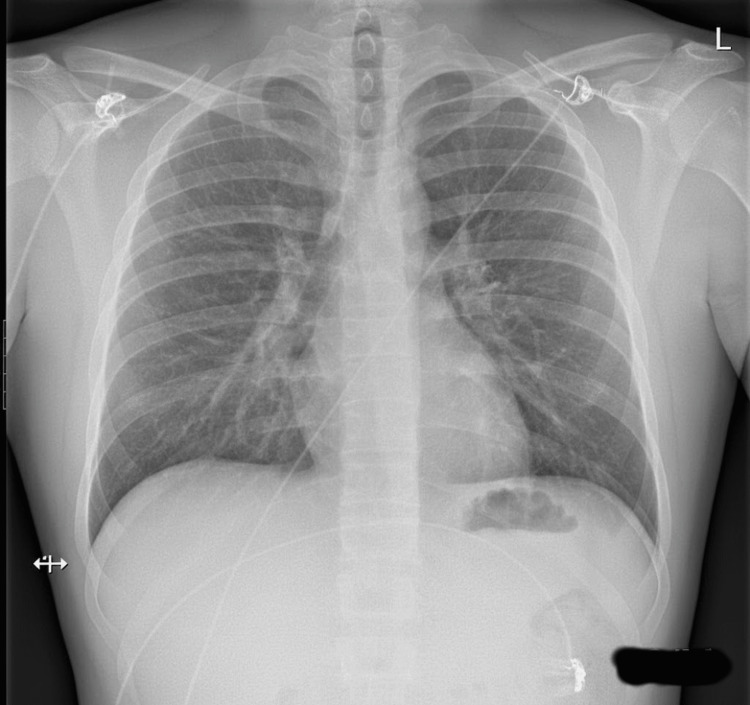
Frontal chest radiograph demonstrating normal cardiopulmonary findings. There is no evidence of pulmonary infiltrates, nodules, hemorrhage, pleural effusion, or mediastinal widening. The cardiac silhouette is normal in size. These findings suggest absence of radiographic pulmonary involvement
